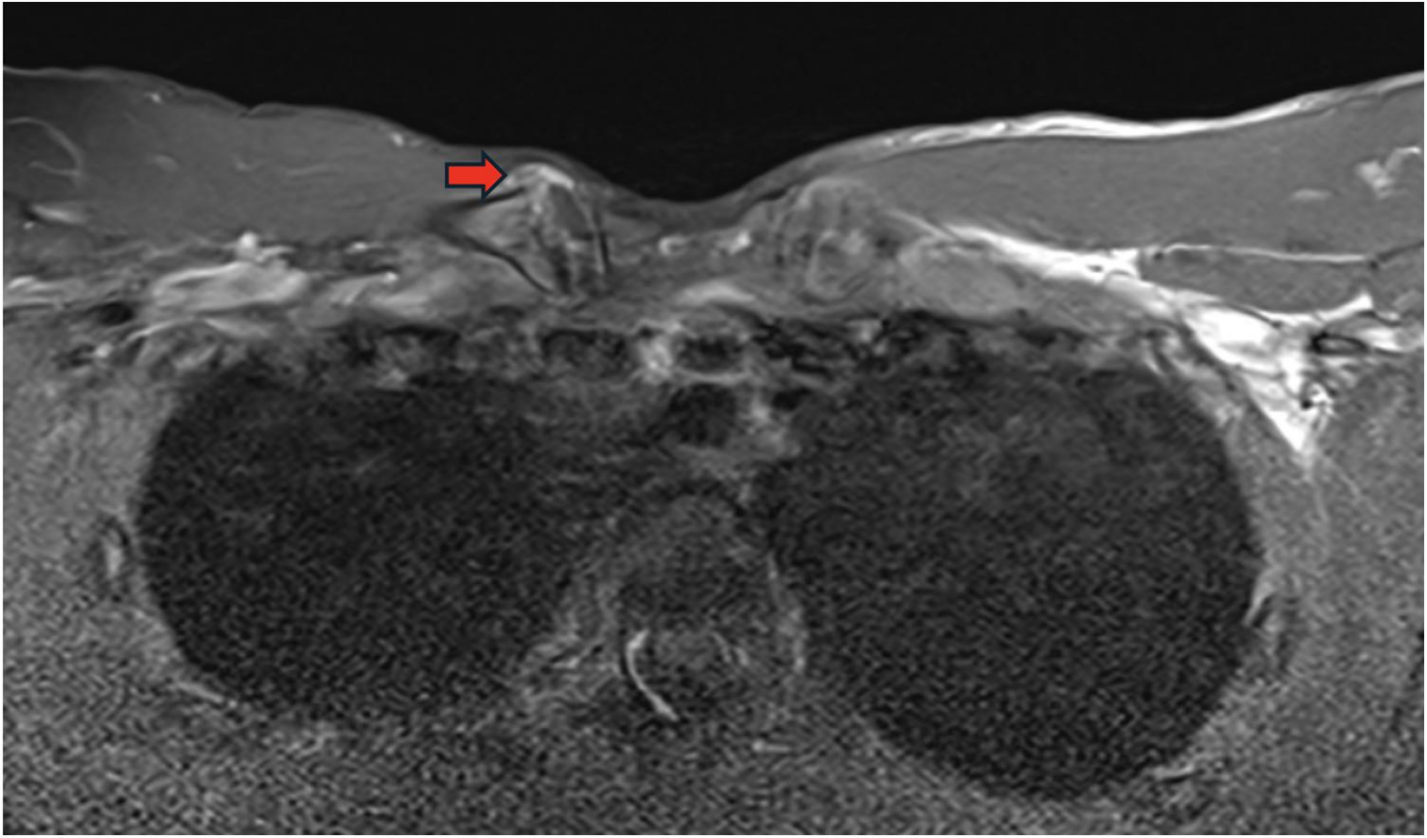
T1-weighted spin echo axial sequence with frequency-selective fat saturation showed an irregularity with widening of the medial physis of the clavicle. There was also an anterior bulging of the sternoclavicular joint capsule suggesting a slight intra-articular effusion (red arrow).
